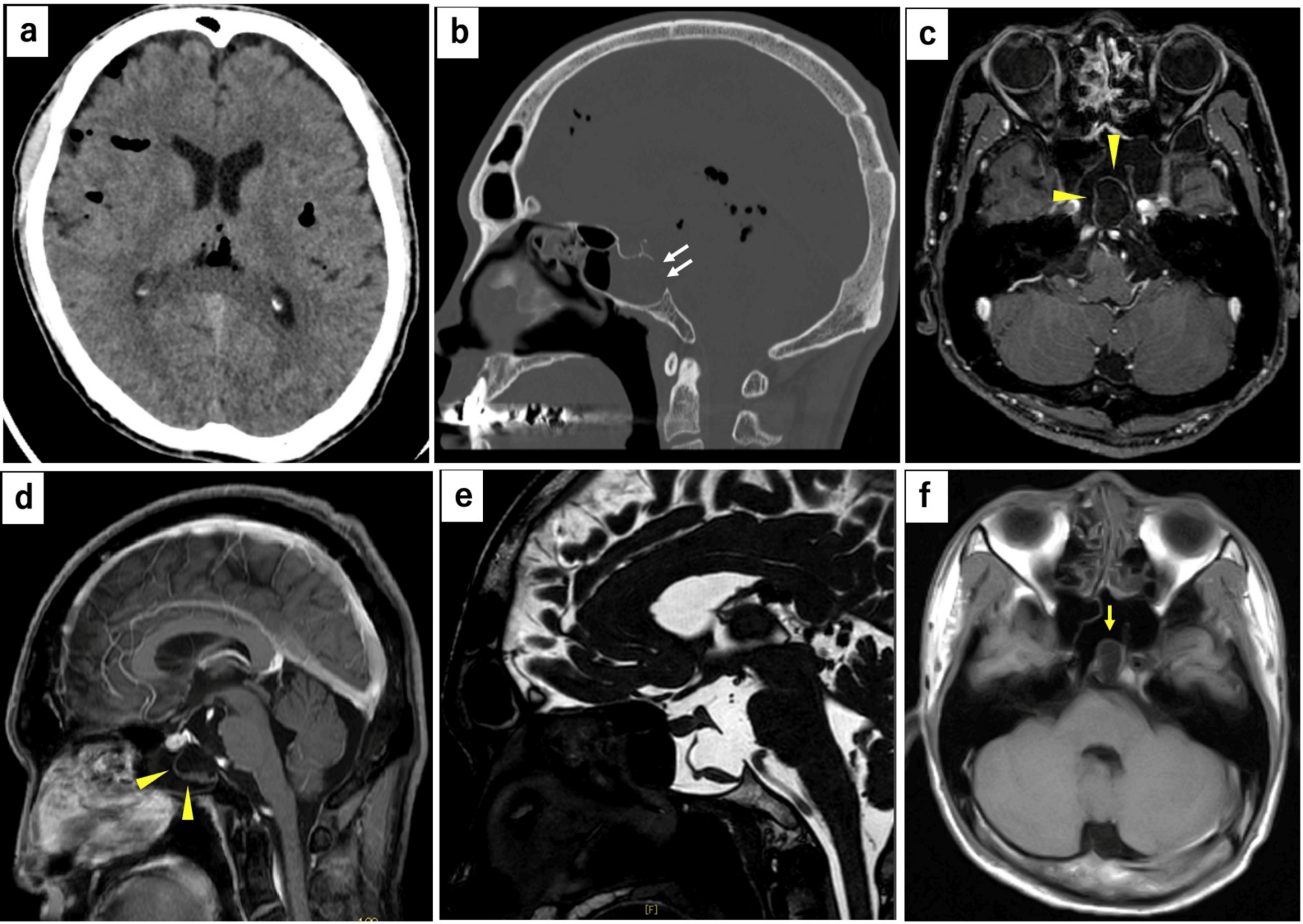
Preoperative CT and MRI findings of clival cystic lesion with cerebrospinal fluid rhinorrhea (a)-(b) CT on admission shows pneumocephalus, a defect of the posterior wall of the clivus (white arrows), and fluid accumulation in the sphenoid sinus. (c)-(e) MRI shows a cyst-like lesion protruding from the prepontine cistern to the sphenoid sinus, not enhanced by gadolinium (yellow arrowheads). (f) MRI at 33 years of age, revealing a cystic lesion in the sphenoid sinus (yellow arrow). CT: computed tomography; MRI: magnetic resonance imaging
